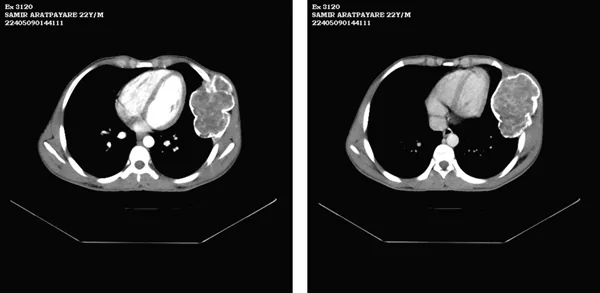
Contrast‐enhanced chest CT cross‐section showing the tumor.
